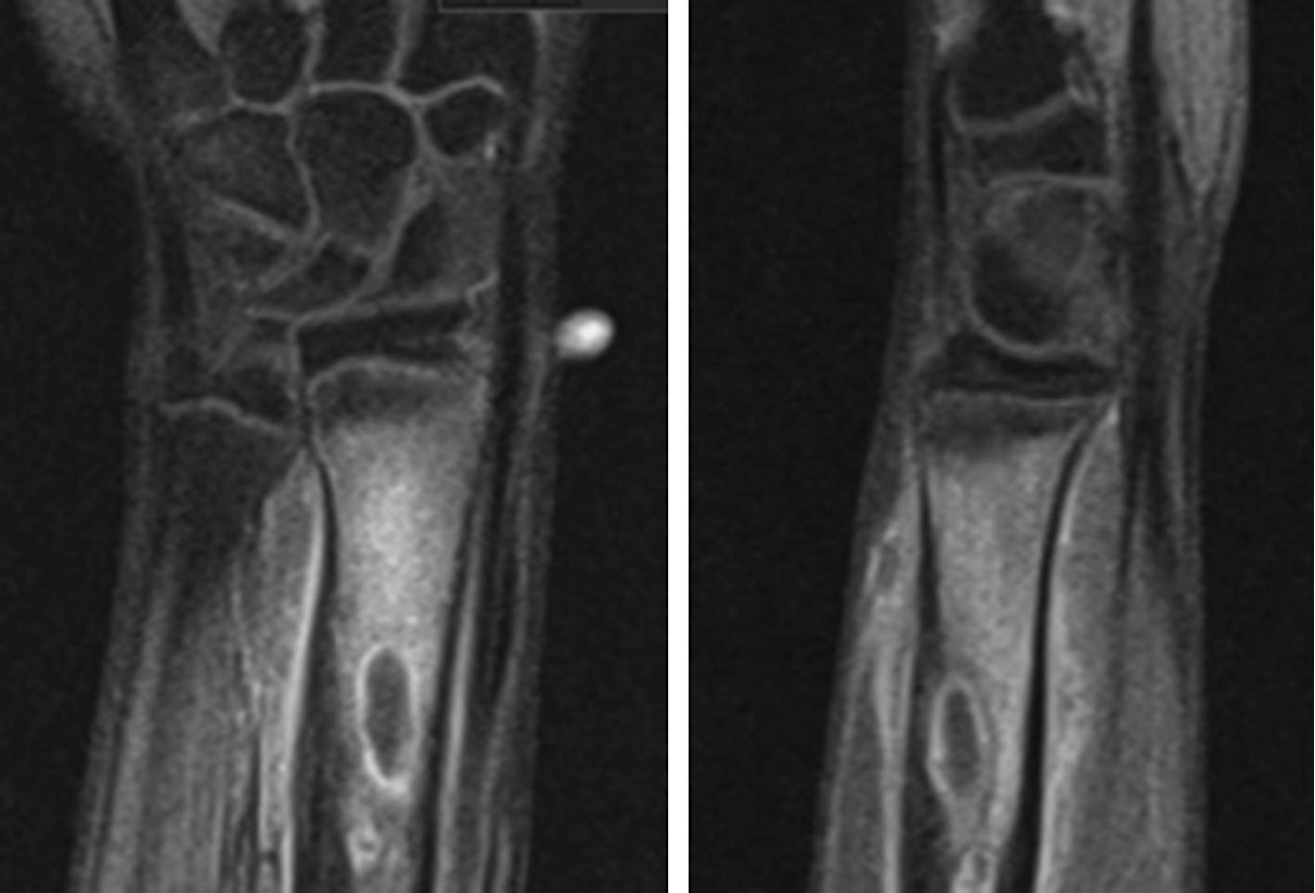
MRI scan of the *left* distal radius showing cystic lesions in the distal radius with surrounding oedema and an area of cortical discontinuity
